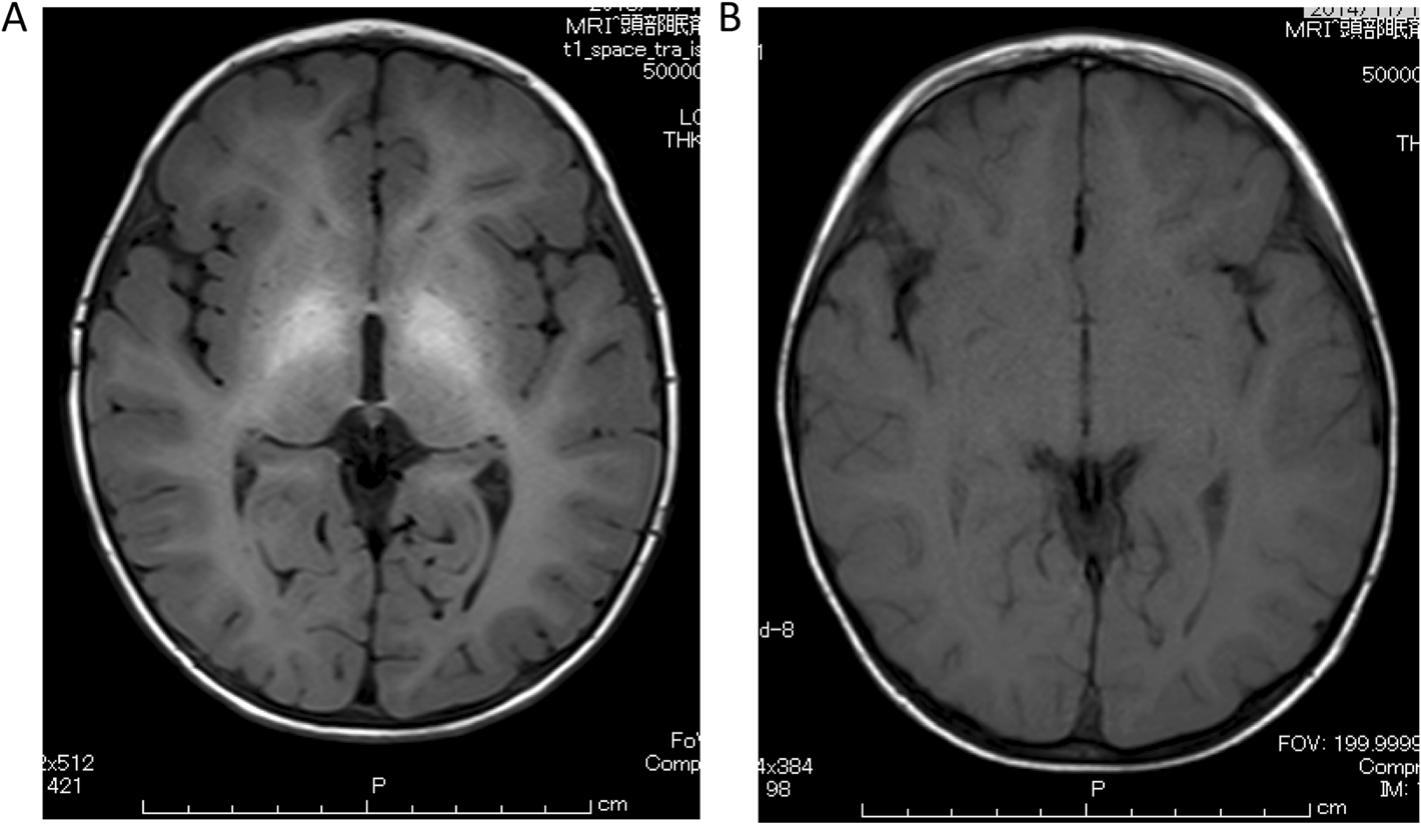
Magnetic resonance imaging of brain. a Magnetic resonance imaging (MRI) of the brain at the age of 1 year and 3 months, revealing high intensity of the pallidum. b MRI at 8 months postoperatively, revealing normalization of the high intensity of the pallidum
